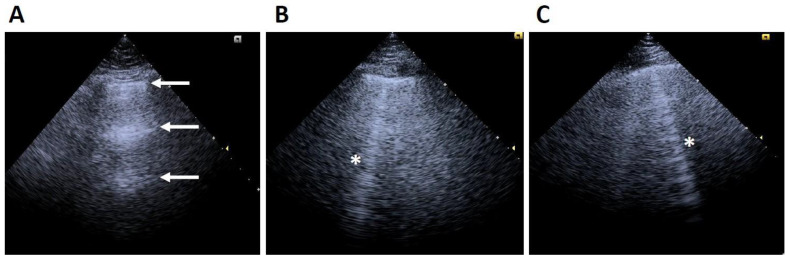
(A) Normal lung ultrasound. Arrows point to normal (A) lines. (B,C) Comet tail artifacts or “B-lines” (*).
